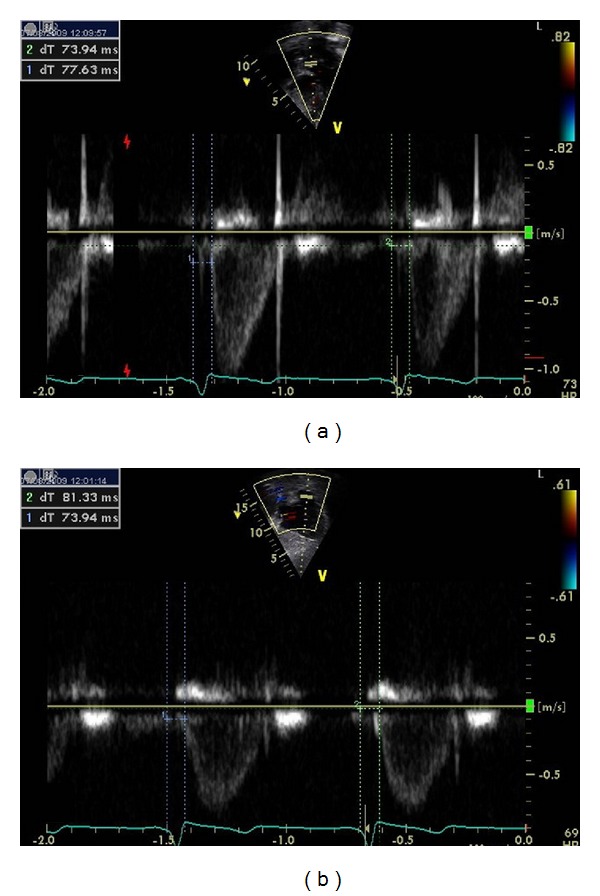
Determination of interventricular mechanical delay (IVMD) using pulsed Doppler mode, corresponding to the difference between aortic (a) and pulmonary (b) preejection delay. No significant delay in left ventricular contraction is observed.
